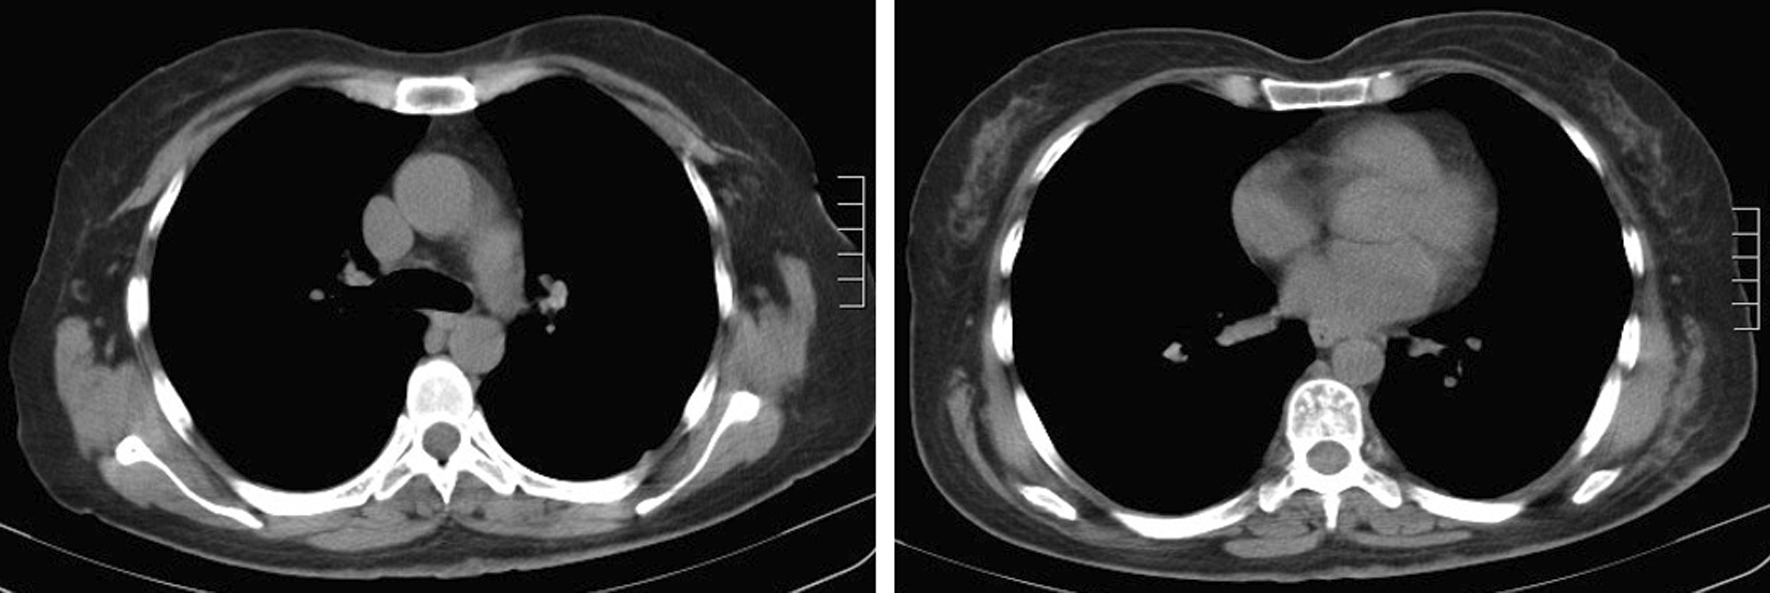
The CT scan of lungs did not show relapse of tumor after 3 months
